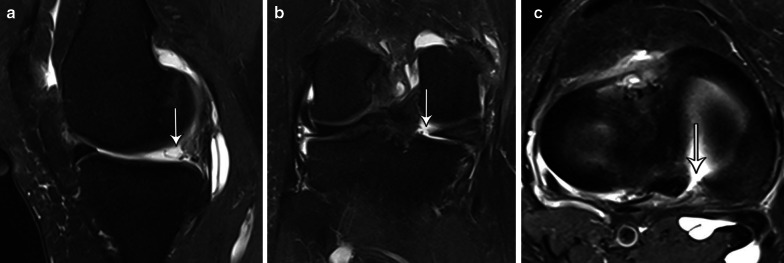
Sagittal (a), coronal (b) and axial (c) T2-weighetd fat-suppressed MR images show “ghost sign” on posterior medial meniscal root attachment (thin arrows). Full-thickness tear of the posterior medial meniscus root is clearly demonstrated in (b) and (c)
